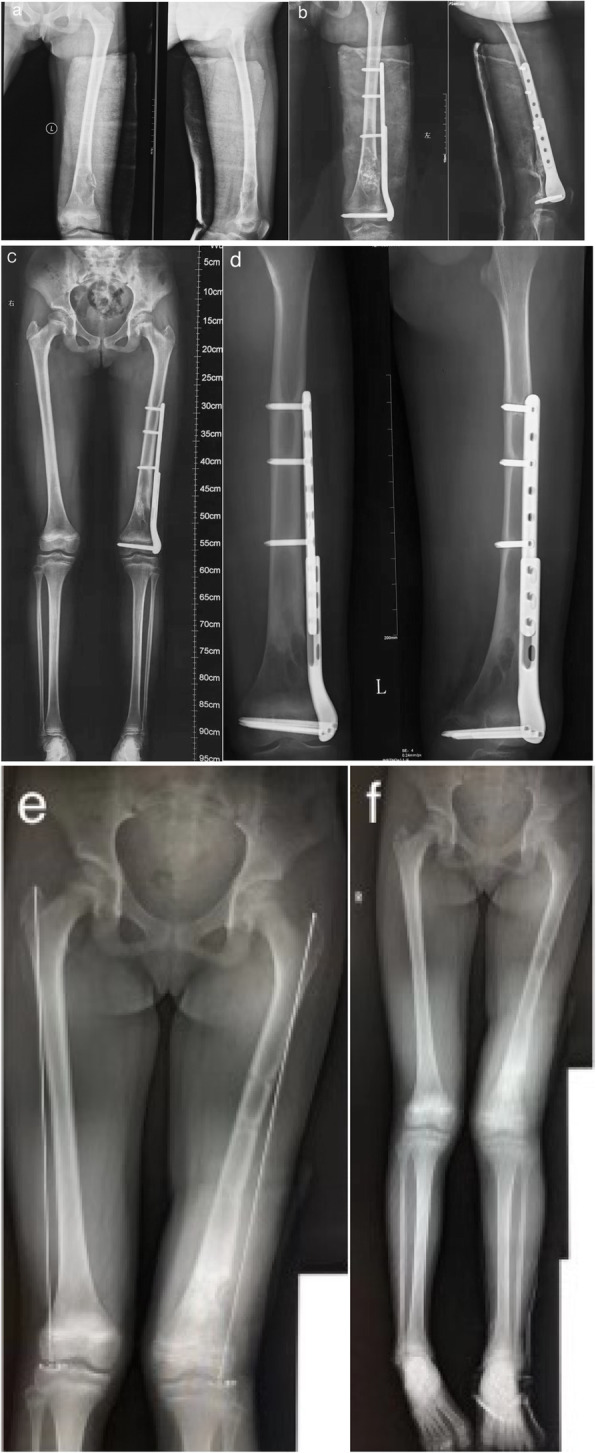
A 12-year-old girl was diagnosed with distal femoral fracture secondary to the unicameral bone cyst and treated with PPSP and bone graft. a Preoperative radiographs on AP and lateral view. b Postoperative radiographs on AP and lateral view. c .Full-length radiograph of lower extremities at 17-month. d AP and lateral radiographs of the femur at 22-month. e AP radiograph of the femur after the plate removal at 25-month. f Full-length radiograph of lower extremities after the plate removal at 25-month
